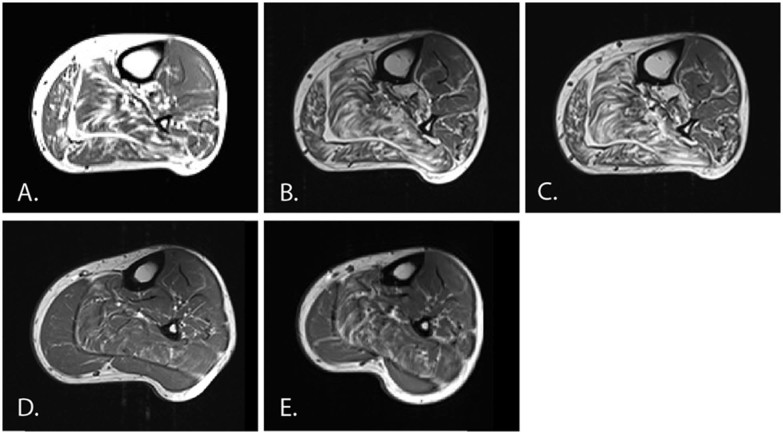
T1 weighted axial MRI images of the lower limbs at baseline, one and two years. A–C are from patient 12 and D–E are from patient 13 (ii). The CMTES and mean calf/thigh Mercuri scores for each image (in parenthesis) are as follows; A, Year 0 (3, 3/1.2); B, Year 1 (7, 3/1); C, Year 2 (3, 3/1); D, Year 0 (0, 0.4/0); E, Year 1 (0, 0.5/0).
